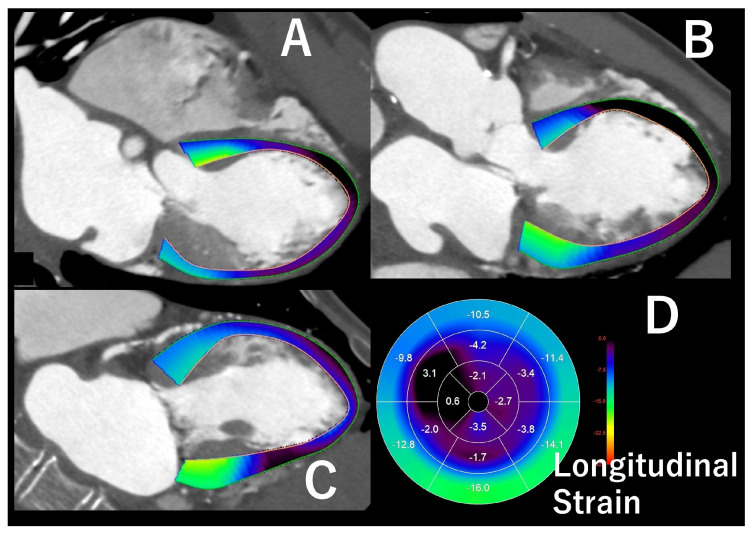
This is a case of takotsubo syndrome. Left ventricular longitudinal strain analysis from cardiac CT performed using a specific software (Ziostation REVORAS v5.3.0.0, Ziosoft Inc., Tokyo, Japan) ((A): four-chamber view used for analysis, (B): three-chamber view used for analysis, (C): two-chamber view used for analysis, (D): left ventricular polar map findings from analysis). CT-based LV longitudinal strain analysis revealed reduced strain in a broad area centered on the left ventricular apex.
